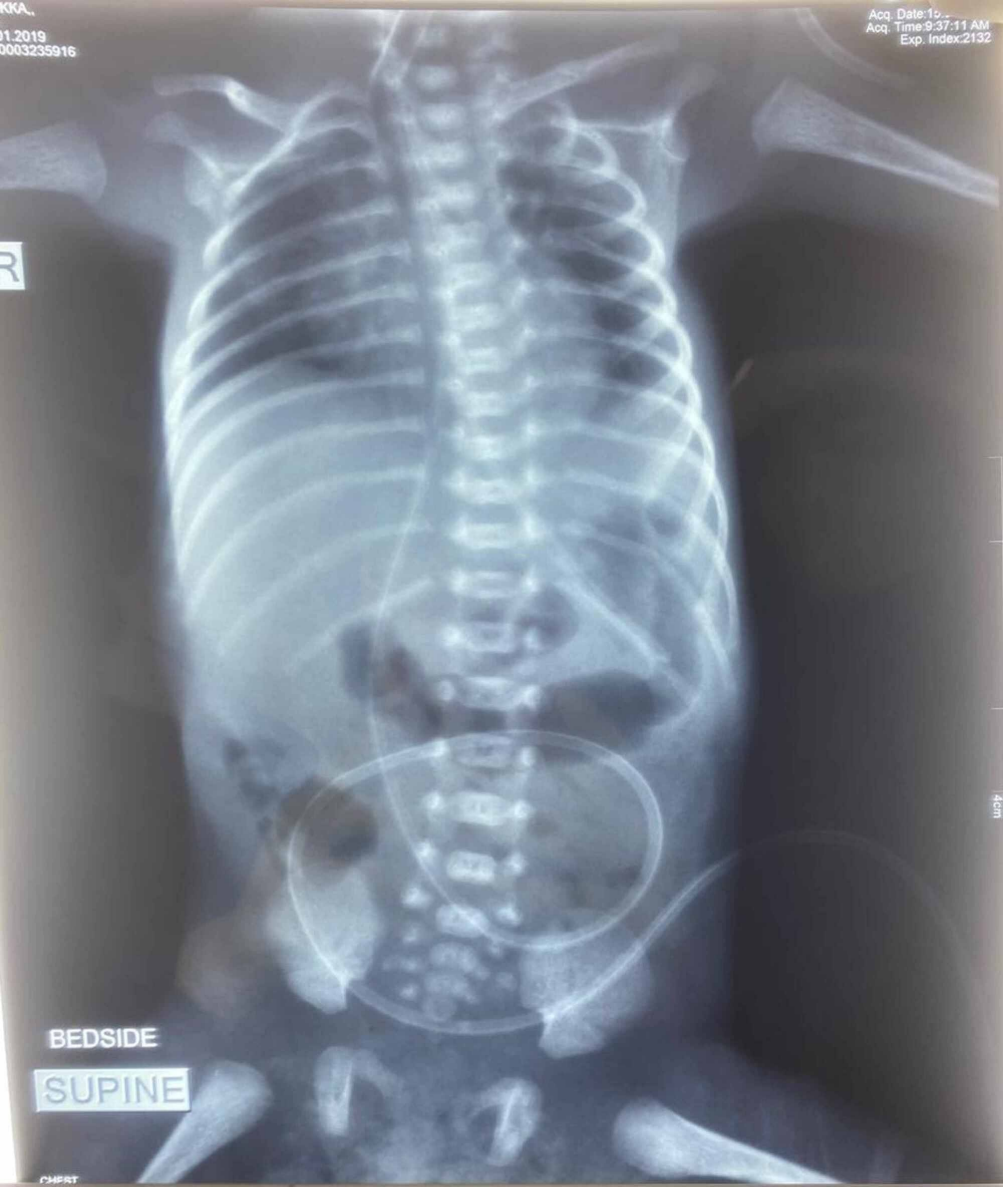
Chest X-ray of the newborn done at one hour of age X-ray chest anteroposterior view with ventilator in situ showing bilateral good air entry.
